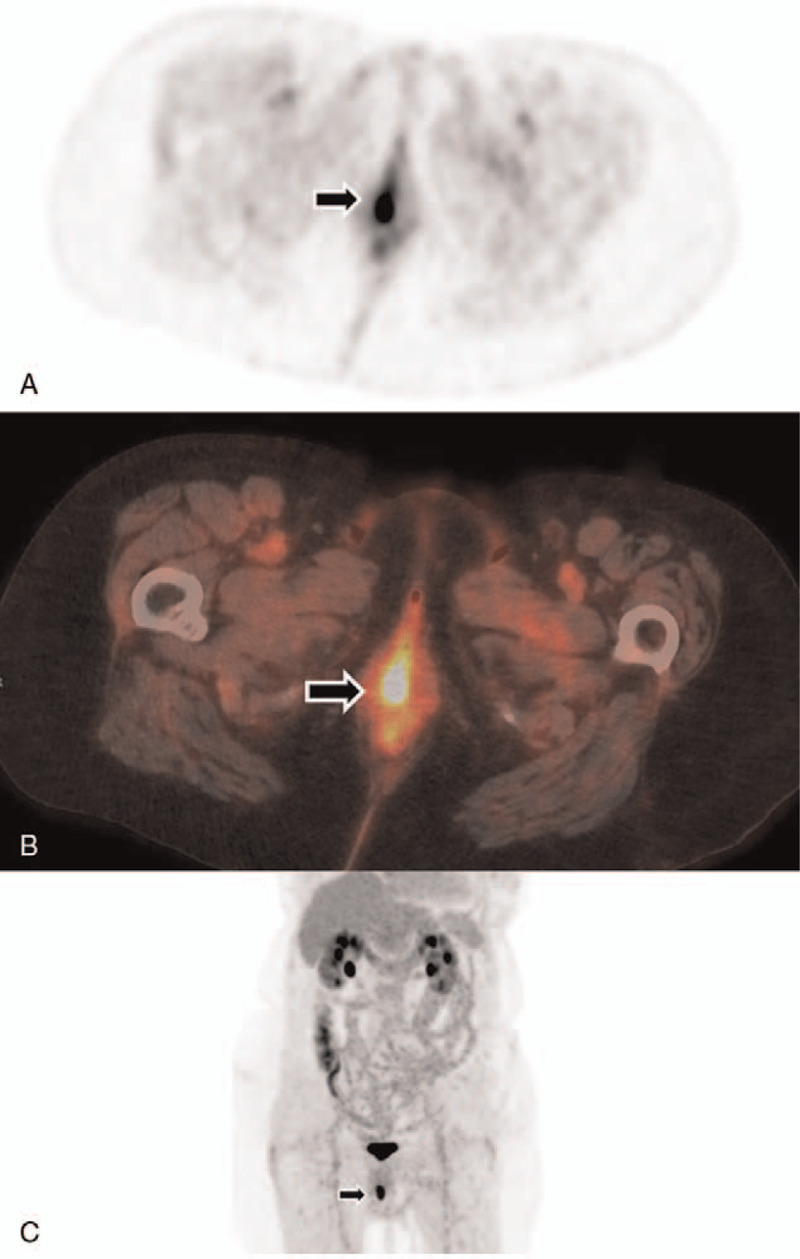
Positron emission tomography/computed tomography (PET/CT) identified a vulvar mass (arrow) with fluorodeoxyglucose (FDG)-avidity (arrow) (A, B, axial scans; C, coronal maximum-intensity-projection).
